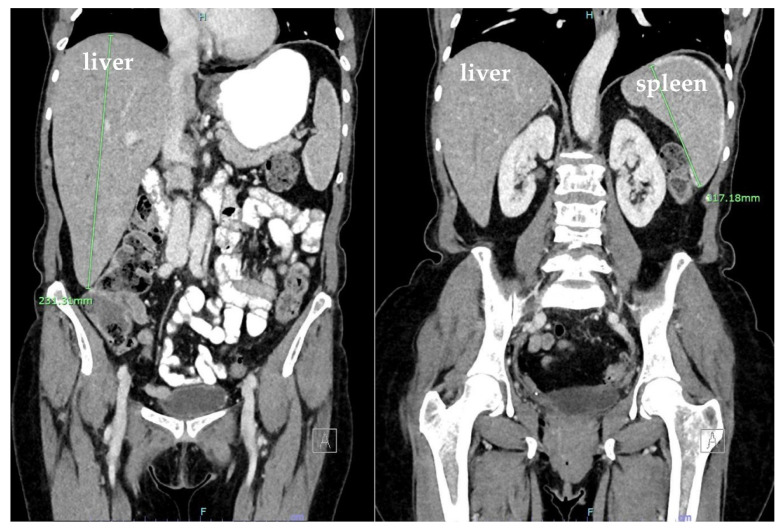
Coronal plane computed tomography (CT) scan with IV contrast of the abdomen and pelvis showing hepatomegaly (23 cm). Spleen was normal.
